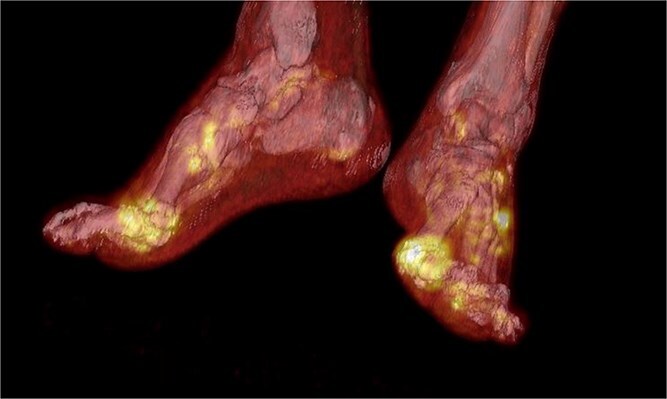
Fusion of 3D volume rendering and MIP of18F-FDG PET-CT demonstrate periarticular tissue swelling and hypermetabolism due to inflammation and arthritic bone errosions in first metatarsophalangeal joint bilaterally.
